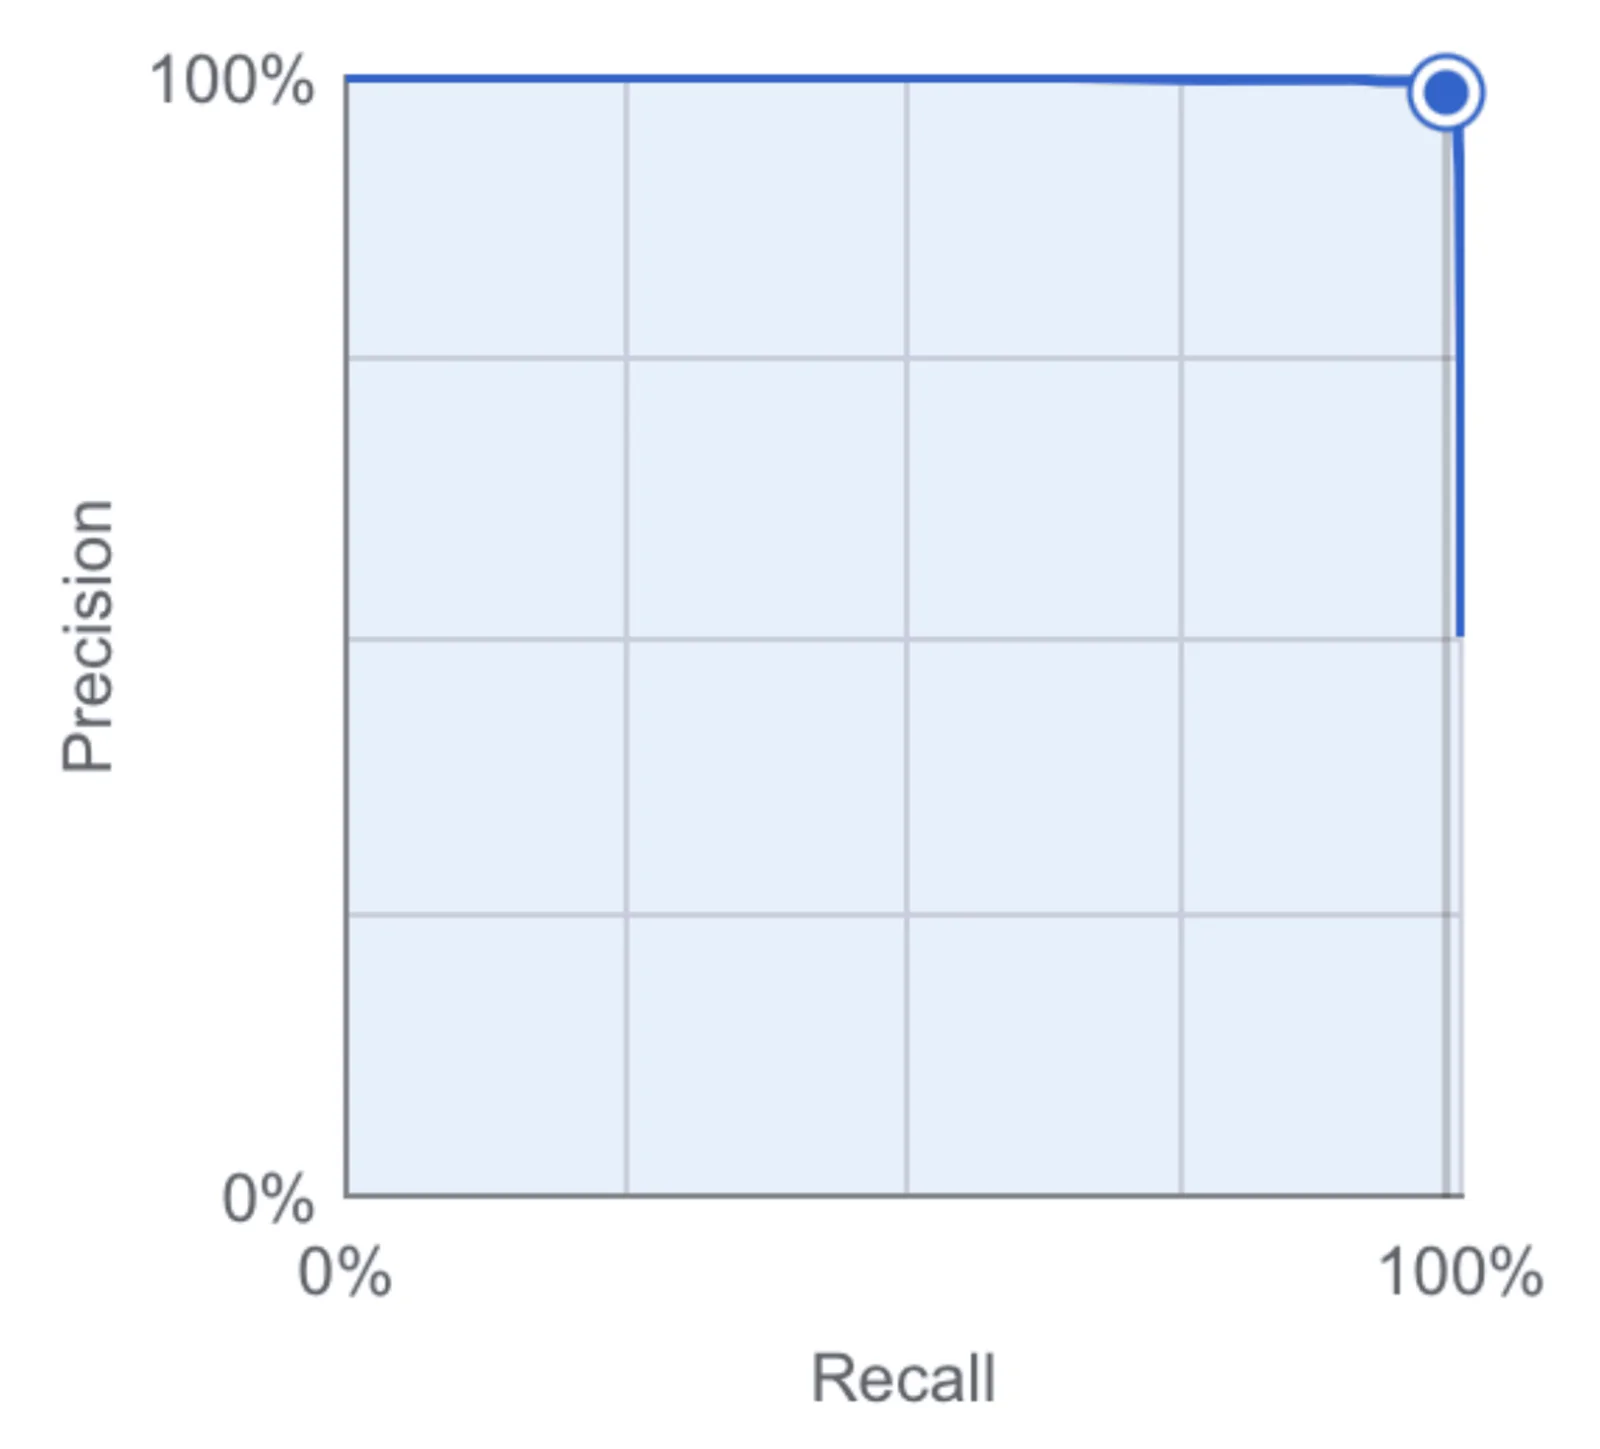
Precision-recall curve for the pediatric pneumonia image-classification model. The precision-recall curve generated by Google Cloud Vertex AI demonstrates consistently high precision across a broad range of recall values. The model achieved an average precision of 0.999, indicating strong discrimination between normal and pneumonia chest radiographs within the internal testing dataset.
